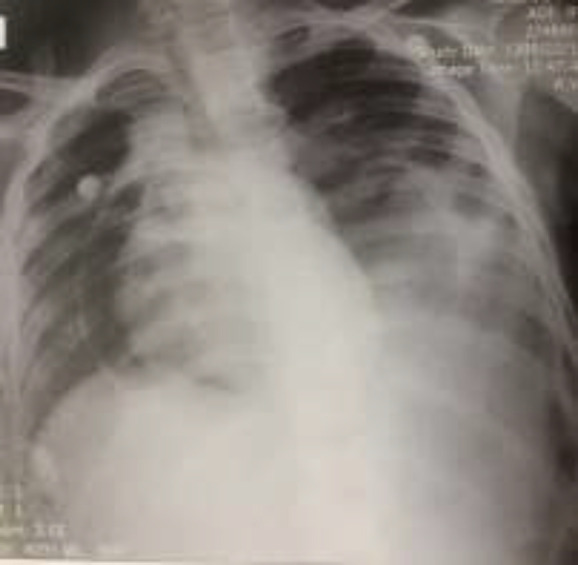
Chest X-ray showing pneumopericardium
